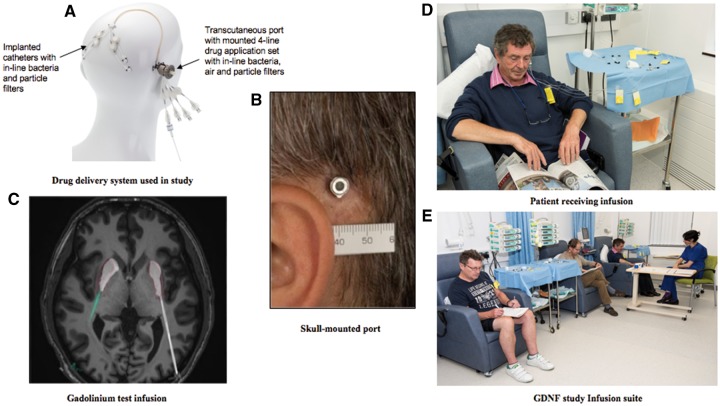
Method of drug administration. (A) Drug delivery system used in the study. A manikin view of the delivery system is shown. Externally, when infusions are delivered, a titanium application set is attached to a skull-mounted port. The application set houses four independent external lines that feed back to four independent B. Braun pumps (not shown) for the administration of GDNF or placebo. Internally, from the skull mounted port, run four independent catheters. (B) The skull-mounted port is the only external component when the patient is not receiving an infusion. (C) Gadolinium test infusion. An axial MRI section at the level of the striatum is shown. Two of the four catheters (dorsal two catheters) can be seen entering either side of the brain posteriorly to penetrate the putamen. T_1_ imaging has been acquired post a test infusion of 2 mM gadolinium down the catheters and into each putamen. Gadolinium can be seen distributed through both putamen from the rostral to caudal extent. (D) A single patient during infusion. (E) Three patients receiving their monthly intraputamenal infusions from B. Braun pumps via their skull-mounted ports, in a standard day-case facility, observed by an accompanying nurse.
